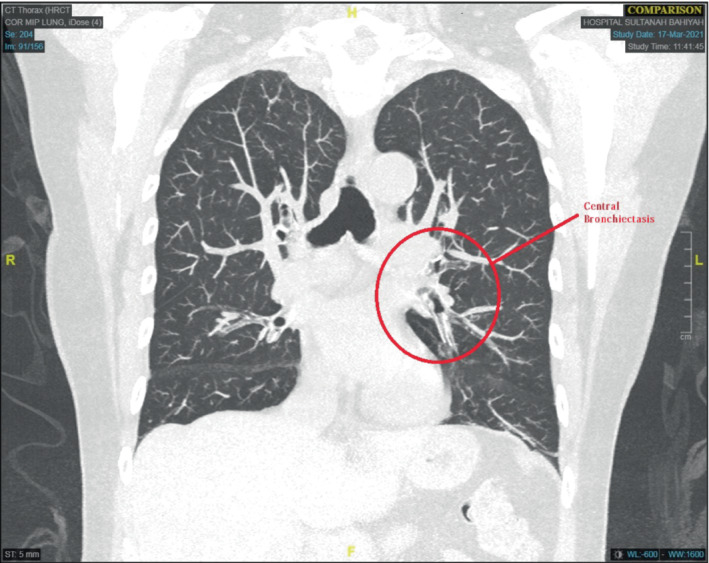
Computed tomography (CT) scan of the thorax, depicting central bronchiectasis due to allergic bronchopulmonary aspergillosis (ABPA).
